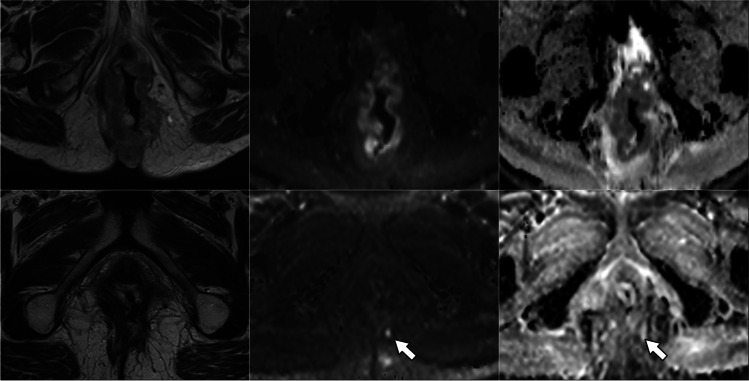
Images in a 64-year-old woman with squamous anal carcinoma. T2-weighted MRI (right column), high *b*-value DWI (middle column) and DWI ADC map (left column). At baseline MRI, tumour of the lower canal staged as T3 (upper row). After treatment (lower row), a small nodule of restricted diffusion in the 7 o’clock position (arrow) lies within a broader area of indeterminate intermediate T2 signal (TRG-3), confirming residual disease (DWI-TRG-4). Incomplete response was recorded clinically
